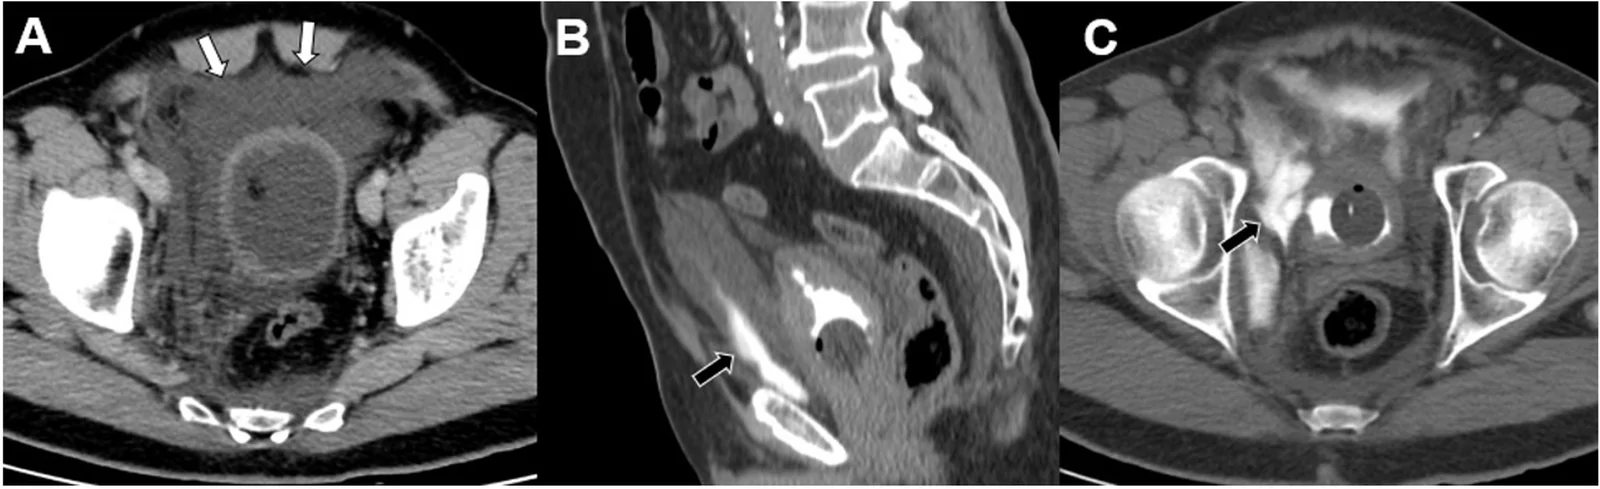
Extraperitoneal bladder rupture into the space of Retzius in a 75-year-old male patient after transurethral resection of the prostate. Axial plane contrast-enhanced CT image (A) shows fluid collection in the space of Retzius (white arrows) and along the right paravesical space. Sagittal (B) and axial (C) plane excretory-phase CT images reveal contrast media extralumination (black arrows) from the bladder into the space of Retzius
